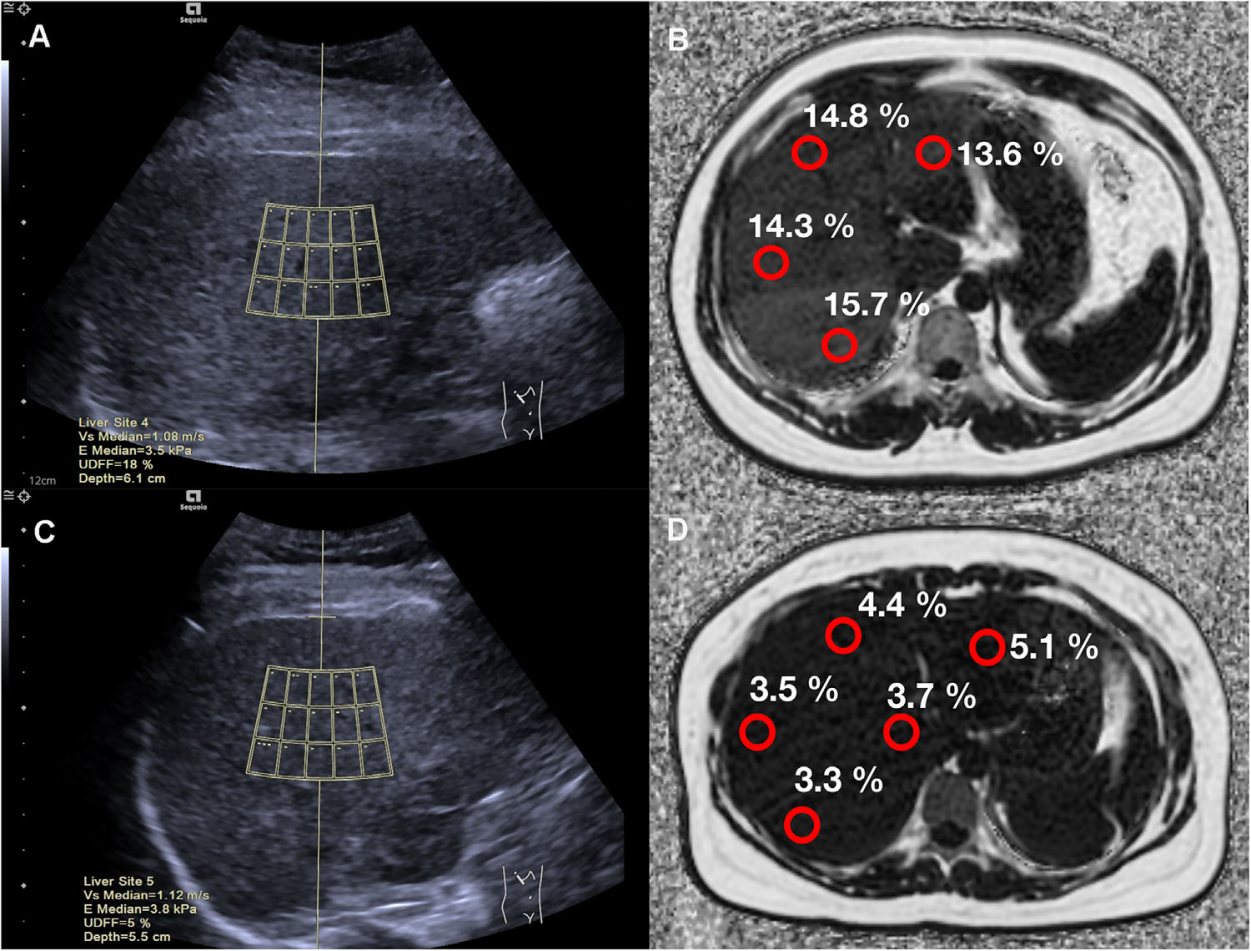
Representative images. (A) A 41‐year‐old female with a body mass index of 29.04 kg/m^2^ and an ultrasound‐derived fat fraction (UDFF) image displayed a value of 18%. (B) The magnetic resonance imaging proton density fat fraction (MRI‐PDFF) image showed a value of 14.8%. (C) A 45‐year‐old female with a body mass index of 24.74 kg/m^2^ and a UDFF image displayed a value of 5%. (D) The MRI‐PDFF image showed a value of 4.3%.
